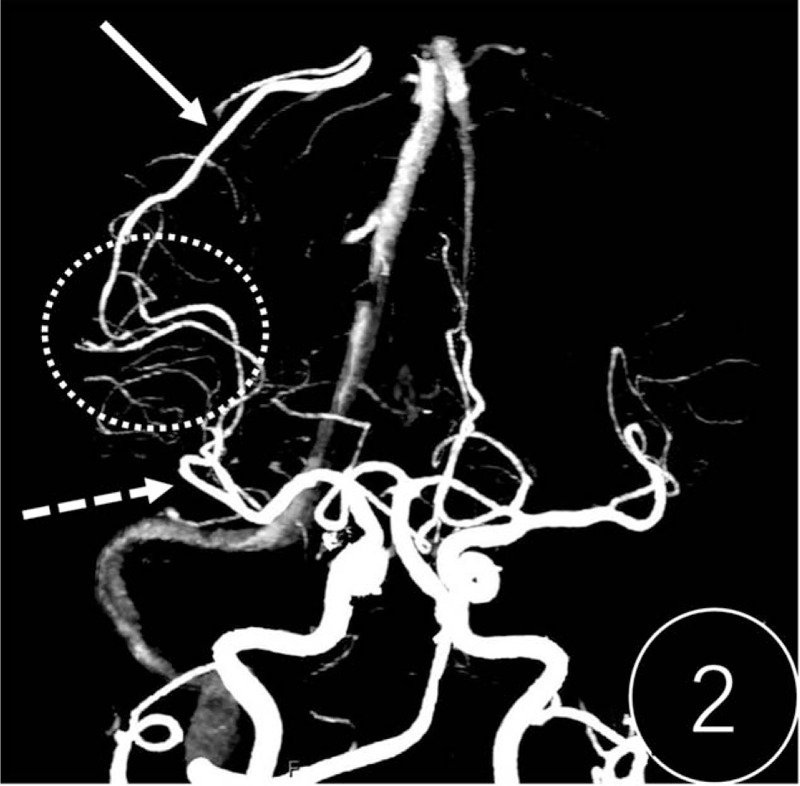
Computed tomography angiography revealed that the feeding artery of the pial arteriovenous fistula (PAVF) was one of the branches of the right middle cerebral artery (virtual arrow). The draining vein (solid arrow) thickened and converged into the superior sagittal sinus. The feeding artery communicated directly with the draining vein (circle area).
